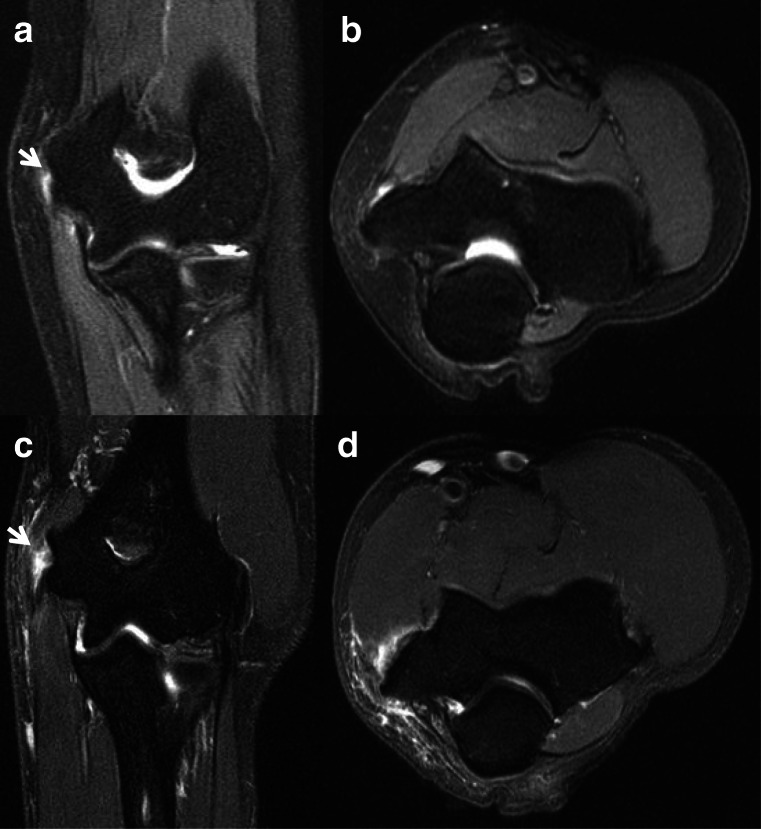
Coronal (a) and axial (b) T2 MR images of the elbow in the same patient demonstrate medial epicondylosis with low-grade partial thickness tears (arrow) and an adjacent UCL sprain. Coronal (c) and axial (d) T2 MR images of the elbow in a different patient demonstrate medial epicondylosis with high-grade partial thickness tears (arrow) and adjacent chronic thickening of the UCL
